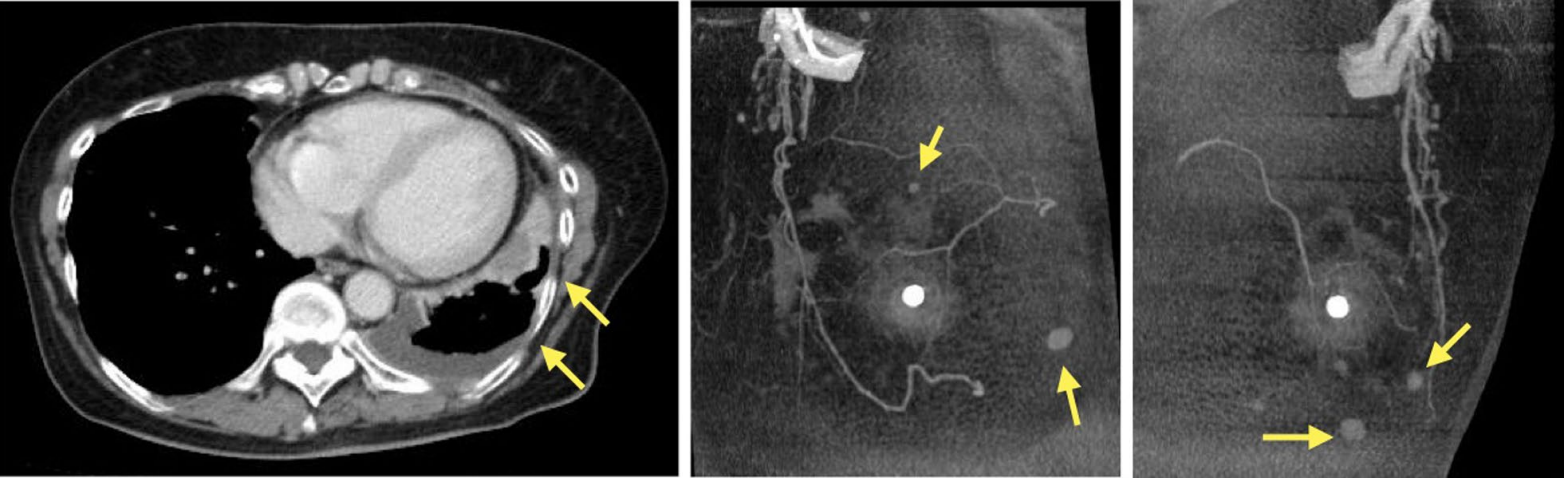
A contrast-enhanced computed tomography (CT) scan revealed irregular pleural thickening and a more tangential mass-like structure at the base of the left lung. Multiple nodules were also revealed in both breasts
